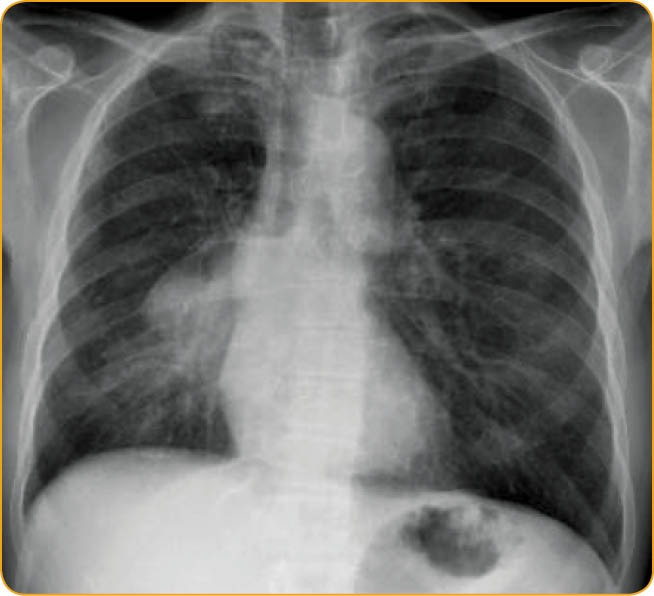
Chest x-ray of patient in Case Study 2.
